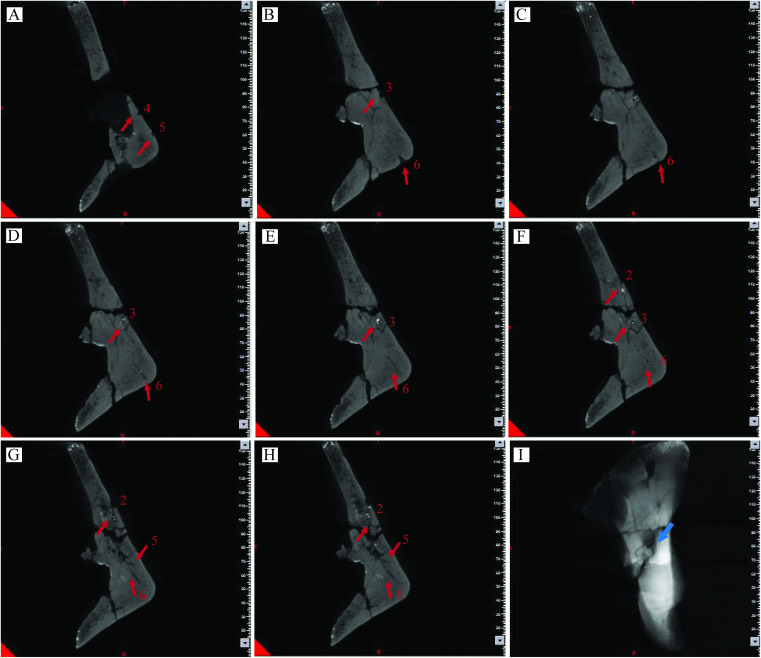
CT scanning of surangular HRS05499. A-H) Sequential images in coronal view (due to crossing angle of perforations). Numbers indicating the perforations correspond to Fig 1A and 1B. Red arrows indicate perforations continuing deep into the bone. I) Rostral view of bone. Blue arrow corresponding to blue arrow on Fig 1B, indicates different types of perforation on which several of the smaller ones (red arrows) connect.
